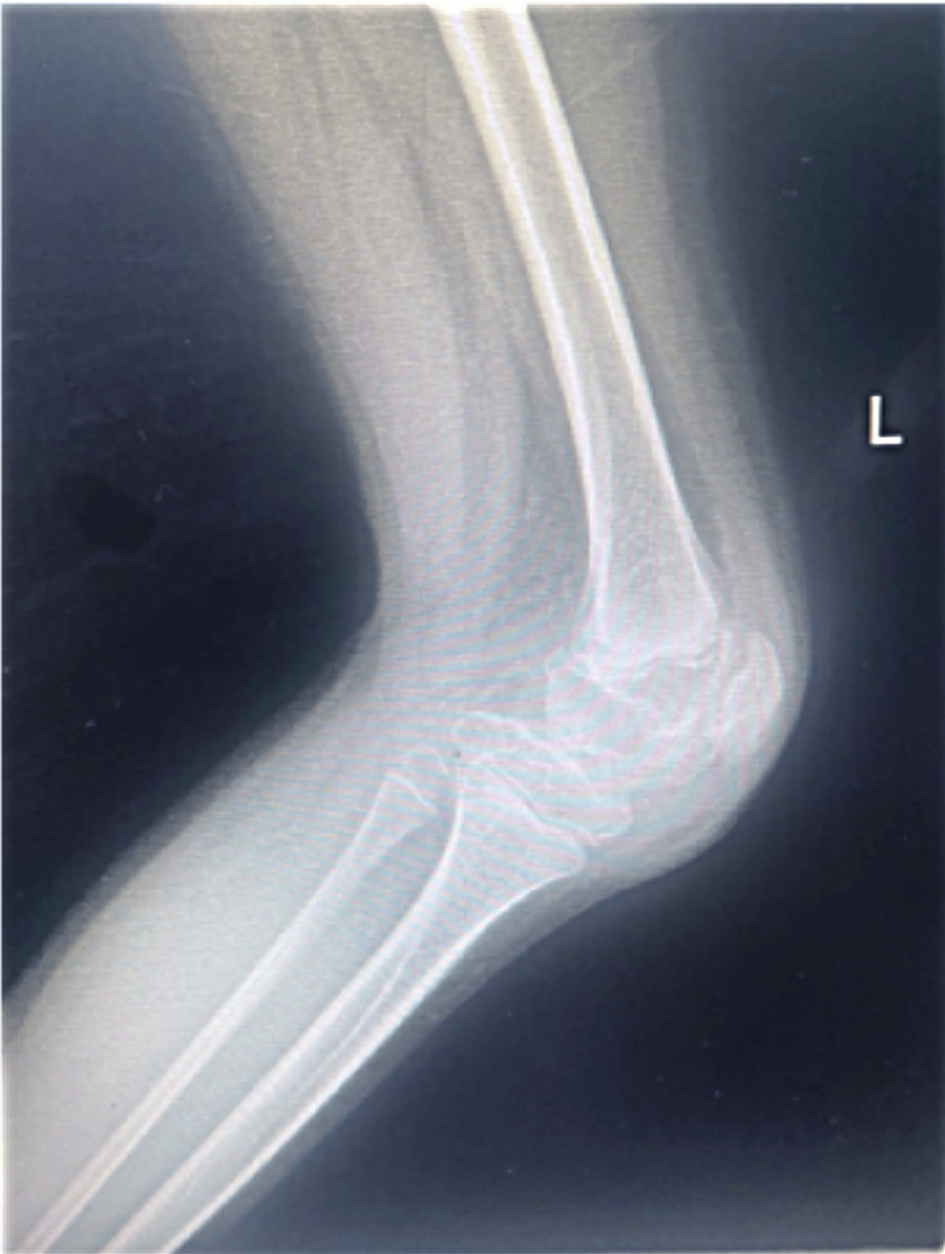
Preoperative lateral X-ray of the left hemophilic knee
